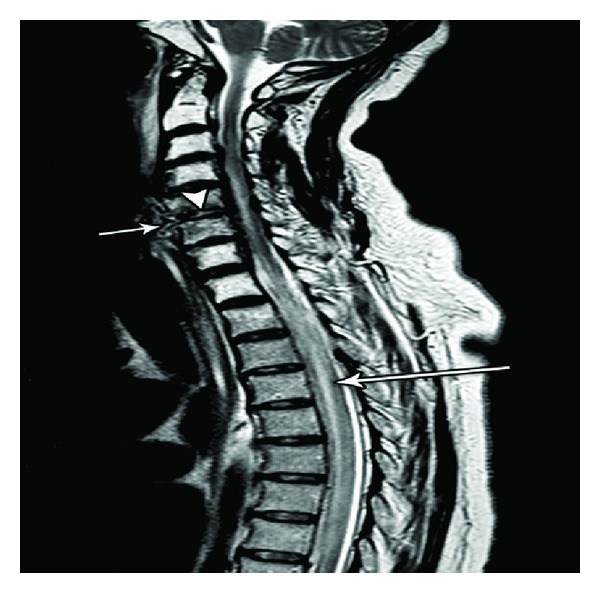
Sagittal T2 MRI demonstrating small collection in the prevertebral space (short arrow) with extension through C5-6 intervertebral disk space (arrow head) and edematous and abnormal high signal of the spinal cord (long arrow).
